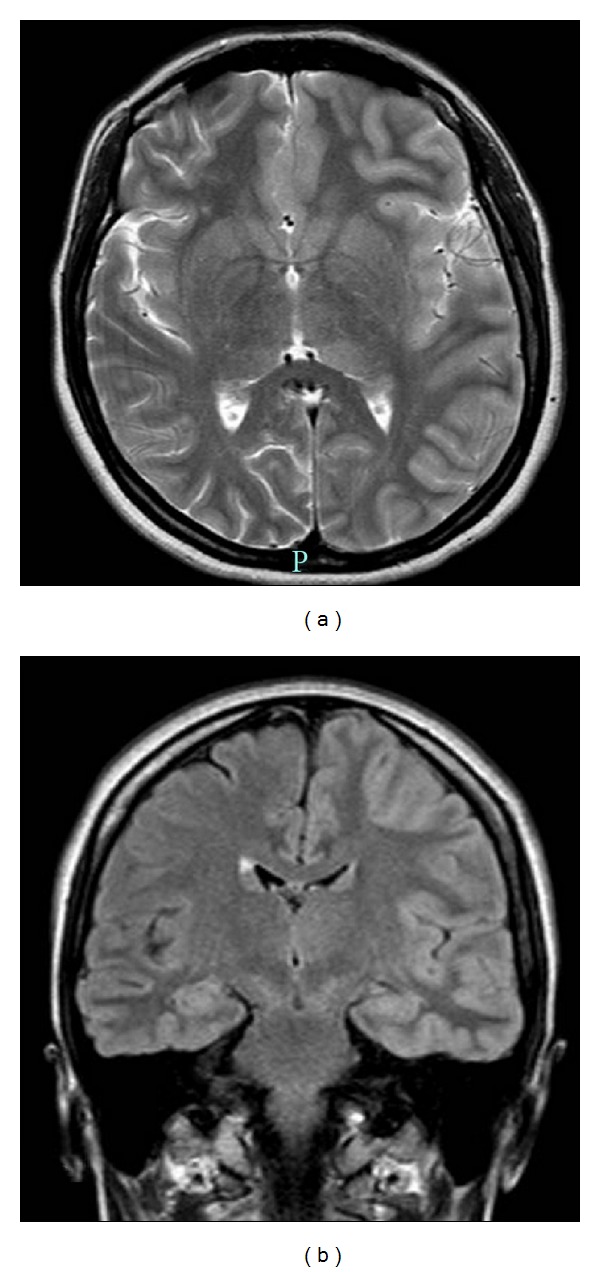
Follow-up MRI of the patient. Coronal fast spin-echo T2-weighted MR images (a) and Flair images (b) through the level of the Sylvian fissures show normal MRI study with disappearance of the previously noted cortical swelling and hyperintensity of the left cerebral hemisphere.
